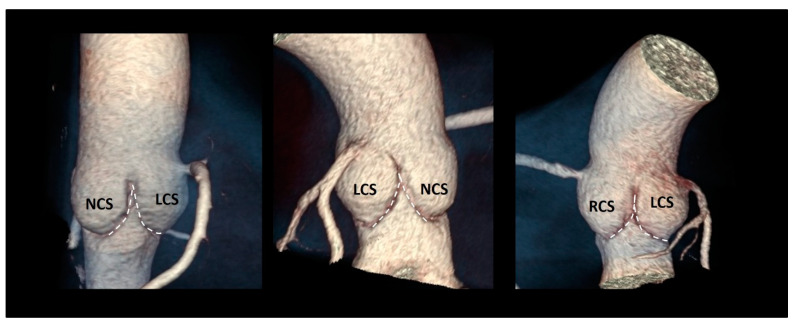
The three inter-leaflets triangles visualized with CT volume rendering format (dotted line). NCS = non-coronary sinus; LCS = left coronary sinus; RCS = right coronary sinus.
